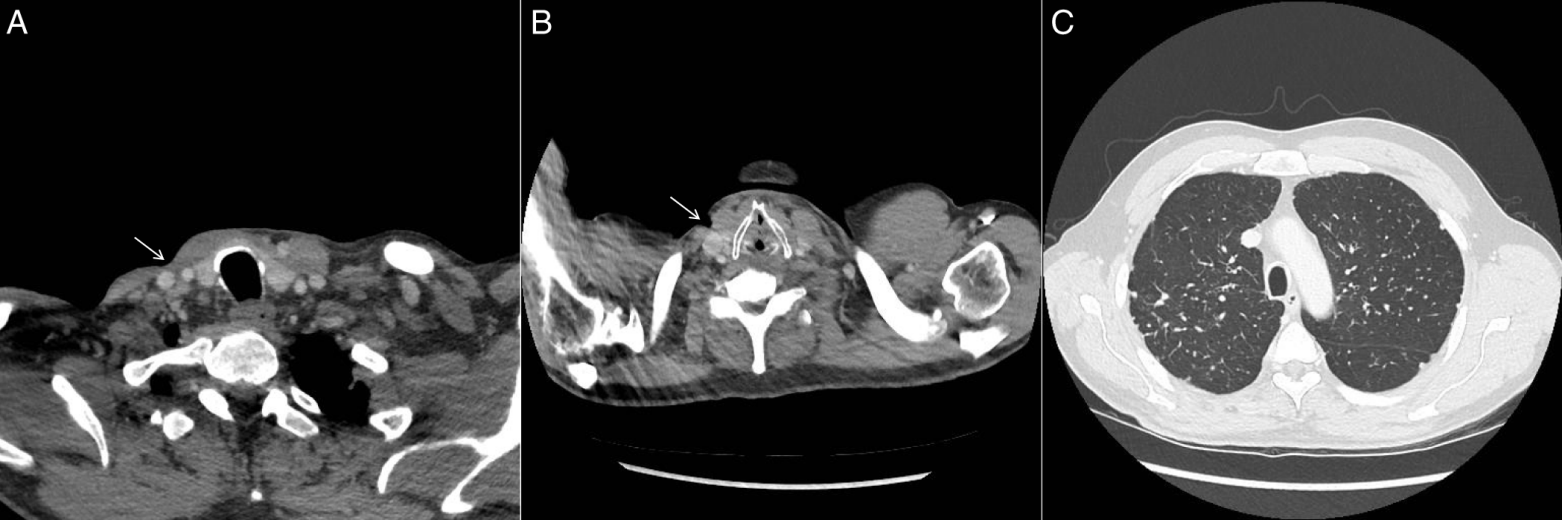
(A,B) Axial computed tomography (CT) scans showing complete resolution of necrotic nodal mass and healing sinus (white arrow) at six and 12 months, respectively. (C) CT thorax at 12‐month follow‐up showing stable pulmonary nodules.
